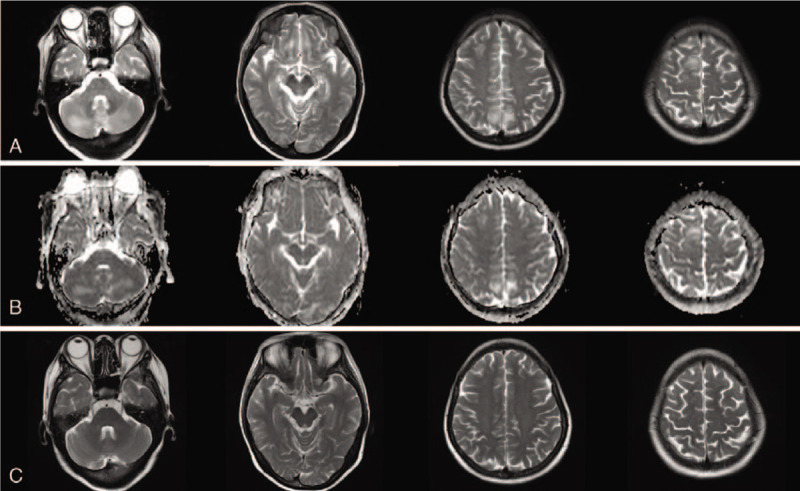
Initial and follow-up cranial MRI. (A, B) Initial cranial MRI on 3rd day after admission showed extensive vasogenic edema in bilateral parieto-occipital lobes and cerebellum. (C) One-month follow-up cranial MRI showed complete resolution of the edematous lesions. MRI = magnetic resonance imaging.
